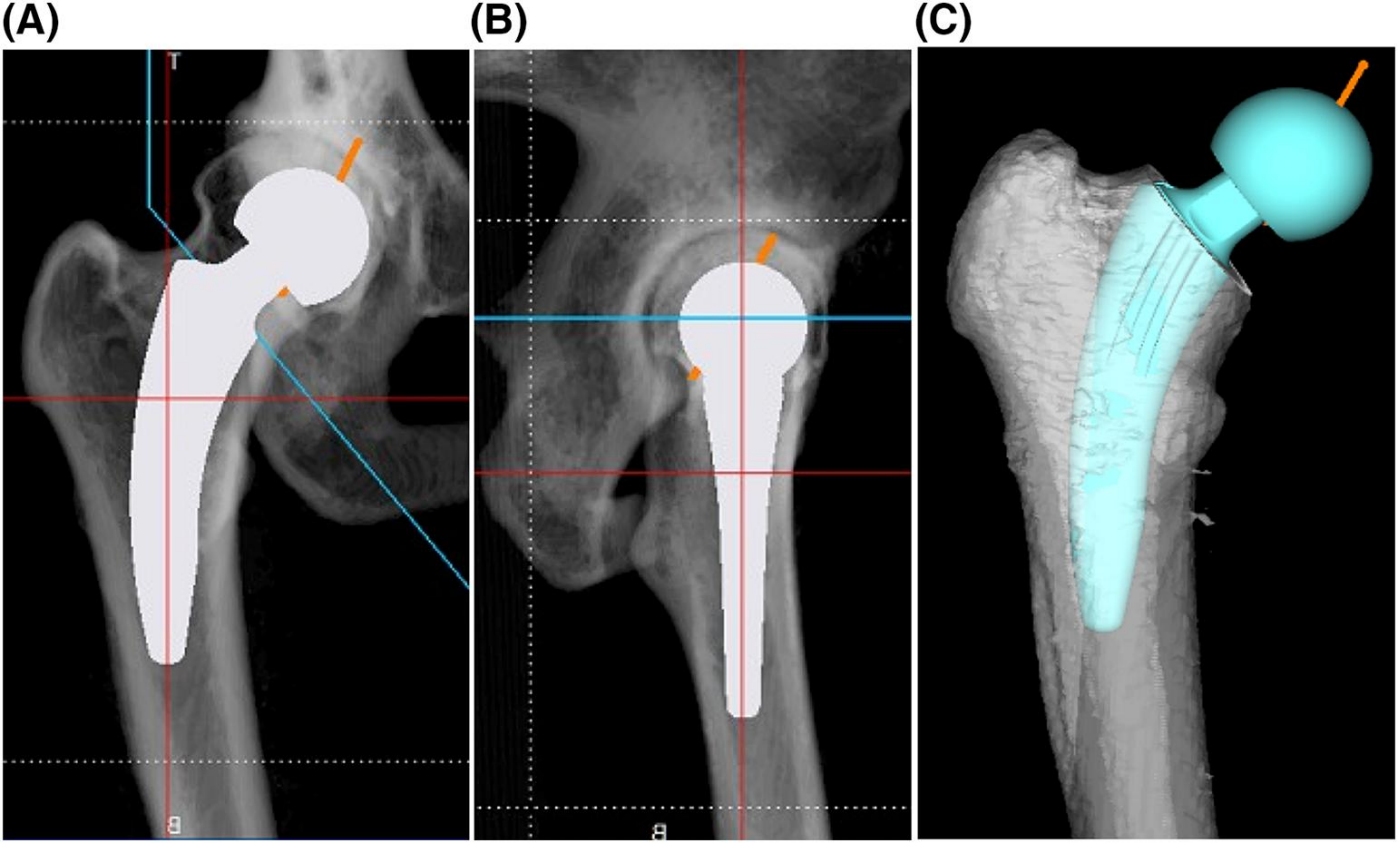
Postoperative radiographs showed varus stem alignment in the anteroposterior view (A) and anterior tilt stem alignment in the lateral view (B). Preoperative three‐dimensional image, anterior view: The MiniHip makes contact with the medial bone cortex proximal to the medullary cavity of the femoral neck and with the lateral bony cortex at the lesser trochanter (C). Owing to the short length and high degree of freedom of placement, the MiniHip prosthesis can reproduce the true centre of the femoral head. A guidance system for positioning the femoral neck‐cut and the angle of insertion of the stem will be useful
